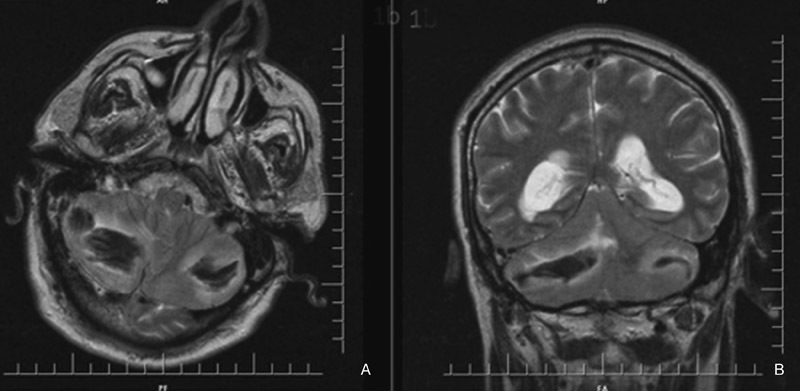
Postoperative T2-weighted cranial magnetic resonance imaging. (A) Axial section and (B) coronal section showing bilateral cerebellar hemorrhage with fourth ventricle compression.
